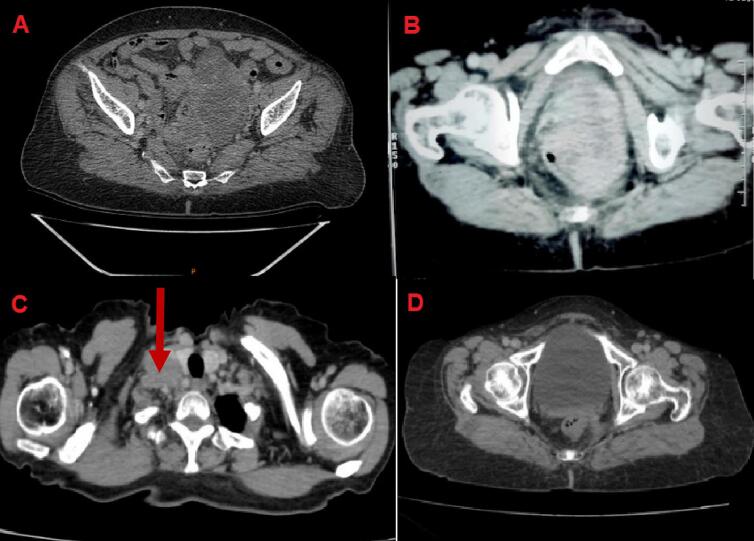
(A) CT scan image of the abdomen showed a left ovary mass, (B) CT scan image showing an irregular circumferential thickening of the rectum, (C) CT scan image of the thoracic-abdominal-pelvic level showing a right supra-clavicular adenopathy, and (D) CT scan image showing complete remission of the primary tumor.
